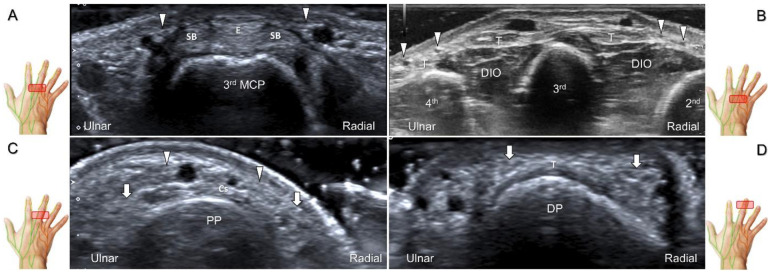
Sonographic imaging of the dorsal common digital nerve on the metacarpal bone (A), toward the metacarpal head (B). Dorsal proper digital nerves on the proximal phalanx (C), and the terminal nerve originating from the palmar proper digital nerve on the distal phalanx of third finger (D). Arrowheads: dorsal common digital nerve; arrows: palmar proper digital nerve. MCP: metacarpal bone; SB: sagittal band; E: extensor tendon; Cs: central slip; PP: proximal phalanx; T: terminal band; DP: distal phalanx; DIO, dorsal interosseous muscle.
